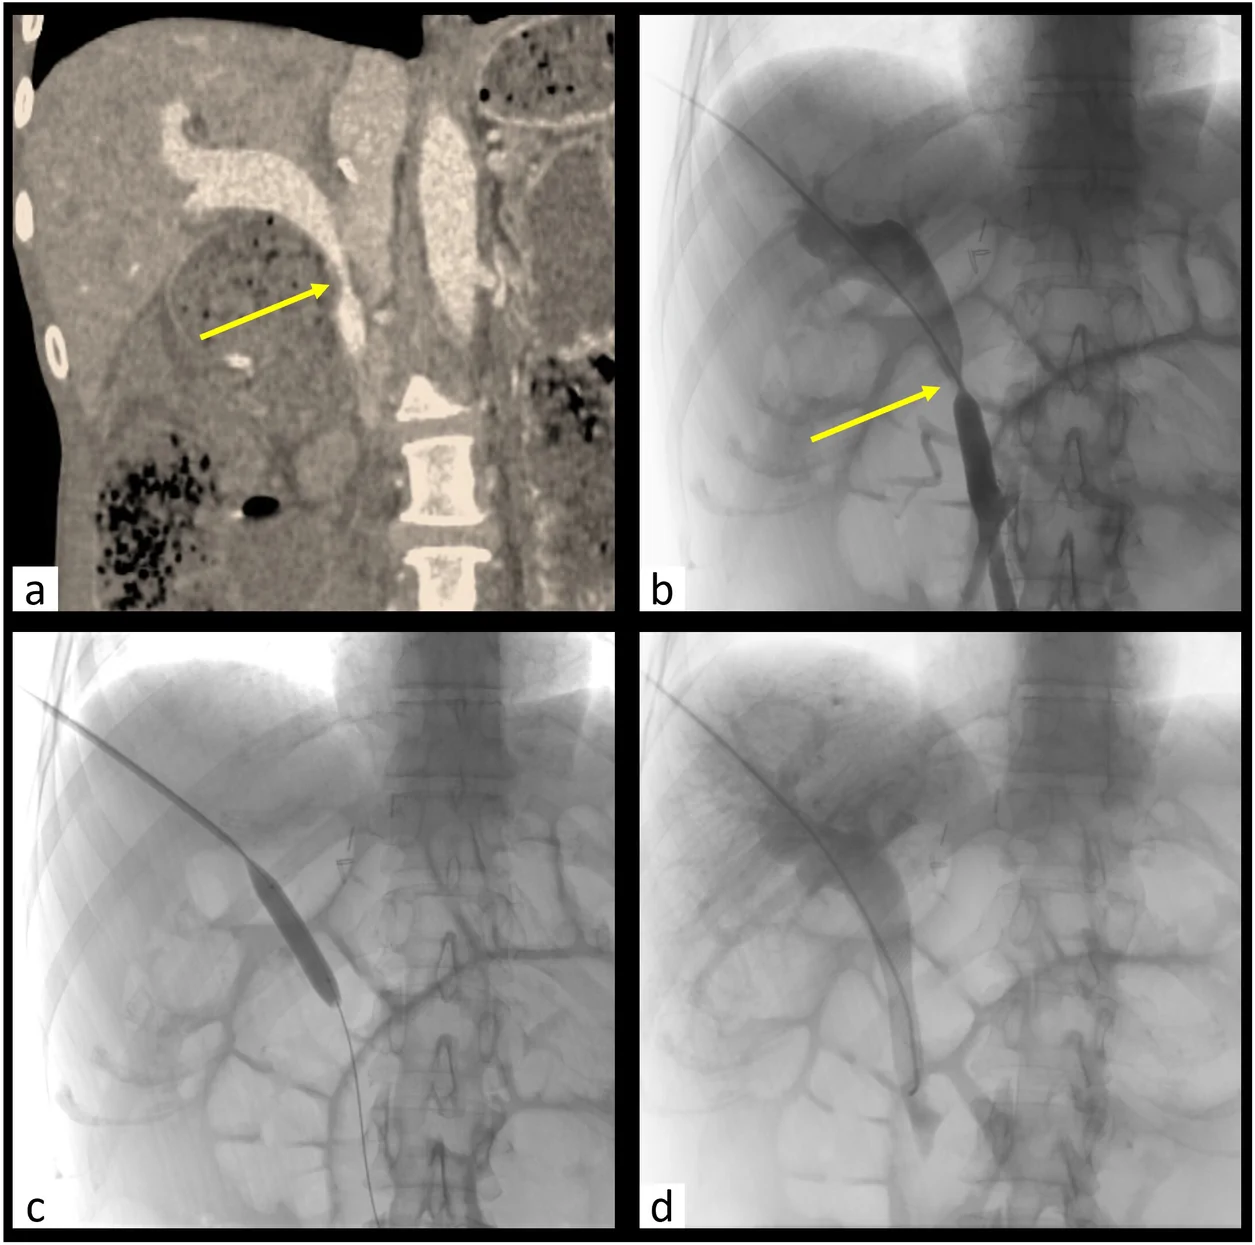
Endovascular management of portal vein stenosis at the shunt-to-portal anastomosis: contrast-enhanced CT (a), pre-intervention portography (b), balloon angioplasty (c), and post-stenting result (d) yellow arrows indicate the site of stenosis.
